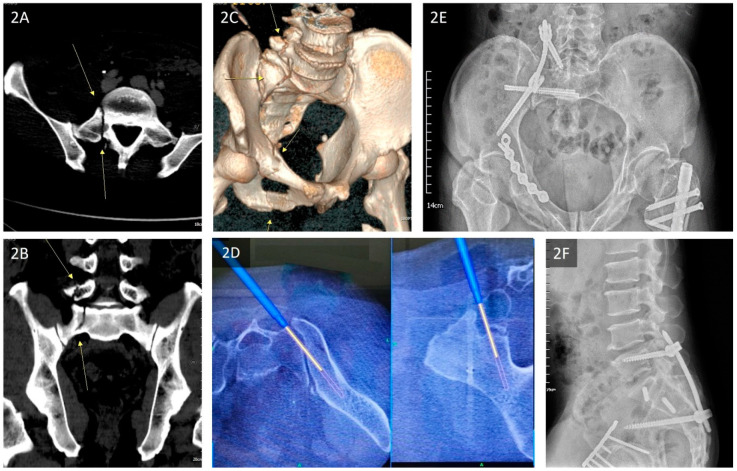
(A–F) Pre- and postoperative radiography and CT images of a 56-year-old man who was hit by a vehicle and had right superior and inferior rami fractures, a right L5 transverse process fracture, and a right sacral fracture with spinopelvic dissociation. The associated injury included a left femoral shaft fracture, right femoral shaft segmental fracture, and left medial malleolar fracture. (A) Axial CT view of the sacrum on admission demonstrating fracture lines (yellow arrows) over the right L5 transverse process; (B) coronal CT view of the sacrum on admission demonstrating fracture lines (yellow arrows) over the right sacrum; (C) 3D CT view of the pelvis showing right superior and inferior rami fractures (yellow arrows), a right L5 transverse process fracture (yellow arrows), and a right AO/OTA 61C1.3 and 54B3 type, Denis Zone II, sacral fracture (yellow arrows); (D) with the assistance of O-arm navigation, the optimal trajectory of the S2AI screw was set (O-arm and Stealth Station S7 Surgical Navigation System, Stryker); (E) postoperative view of the inlet pelvis—the sacral fracture was stabilized by triangular osteosynthesis with S2AI screws, and the right superior rami fracture was reduced and fixed with a pre-contoured locking plate; (F) postoperative lateral view of the sacral spine. Abbreviations: CT = computed tomography.
